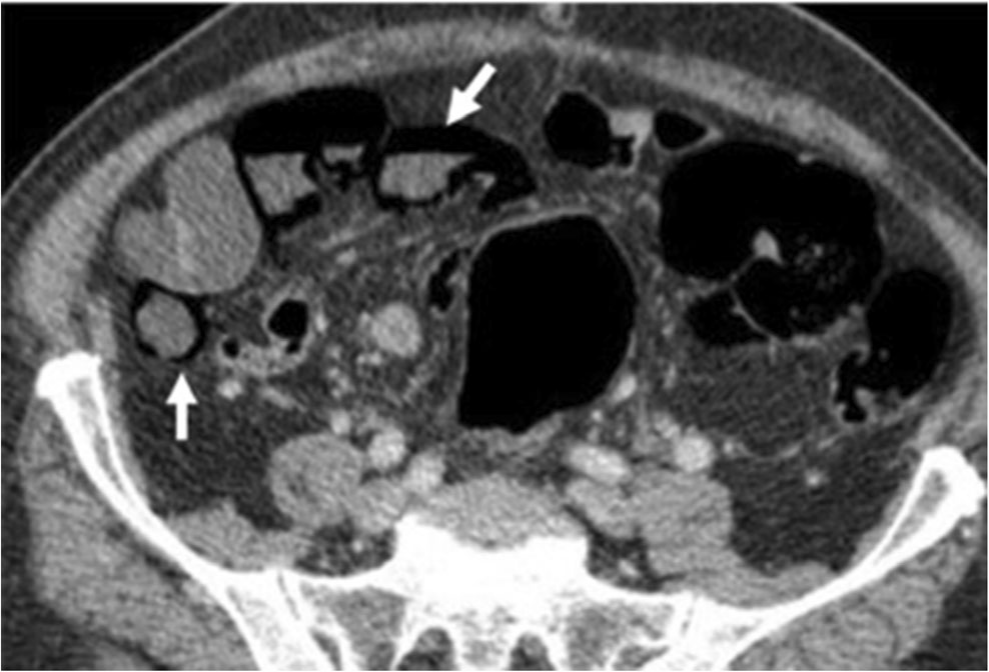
Pneumatosis. A 91-year-old male with mesenteric infarction. Contrast-enhanced CT images of the lower abdomen show gas in the bowel wall (white arrow)
